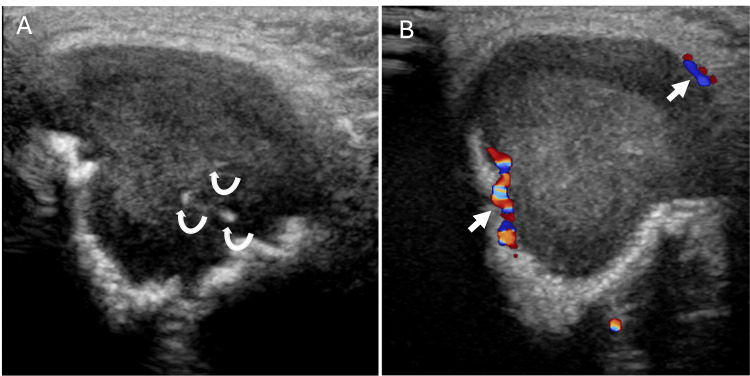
Targeted grayscale (A) and color Doppler (B) sonographic evaluation of the area of clinical concern demonstrates a solid mass with few internal punctate echogenic foci (curved arrows), likely calcifications, and minimal peripheral vascularity on color Doppler (arrows).
